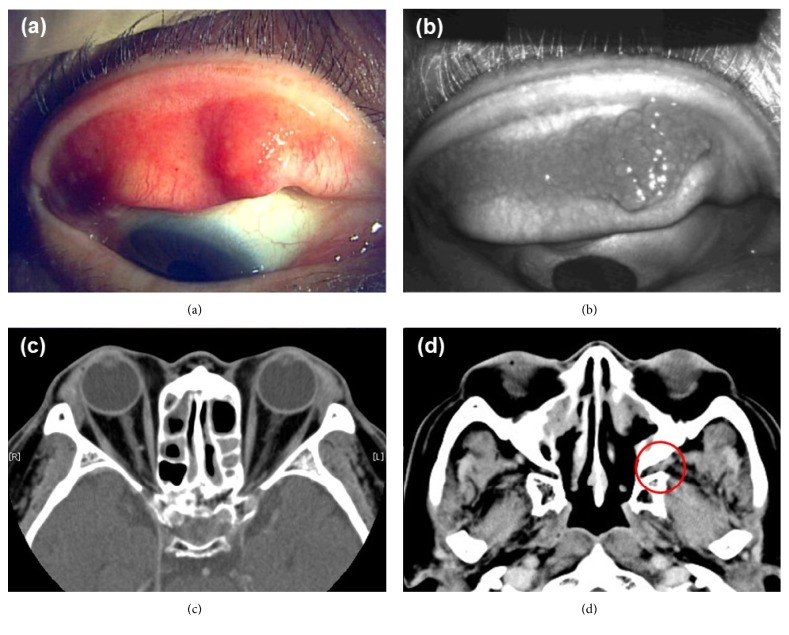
Clinical examination and computed tomography (CT). (a) Color photo and (b) scanning laser ophthalmoscope image showing papillary of right upper eyelid. (c) Orbital CT showing mild swelling of bilateral lacrimal glands. (d) Orbital CT showing hypertrophy of soft tissue in left pterygopalatine fossa (red circle: left pterygopalatine fossa).
